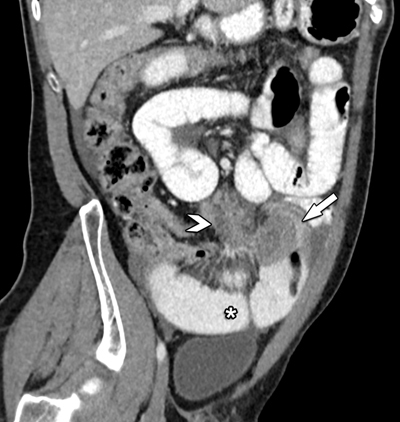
Oblique coronal view of contrast-enhanced CT shows the close relation between the mesenteric mass (arrowhead) and its extension towards the small bowel (arrow). Note the distended small bowel loops (*).
